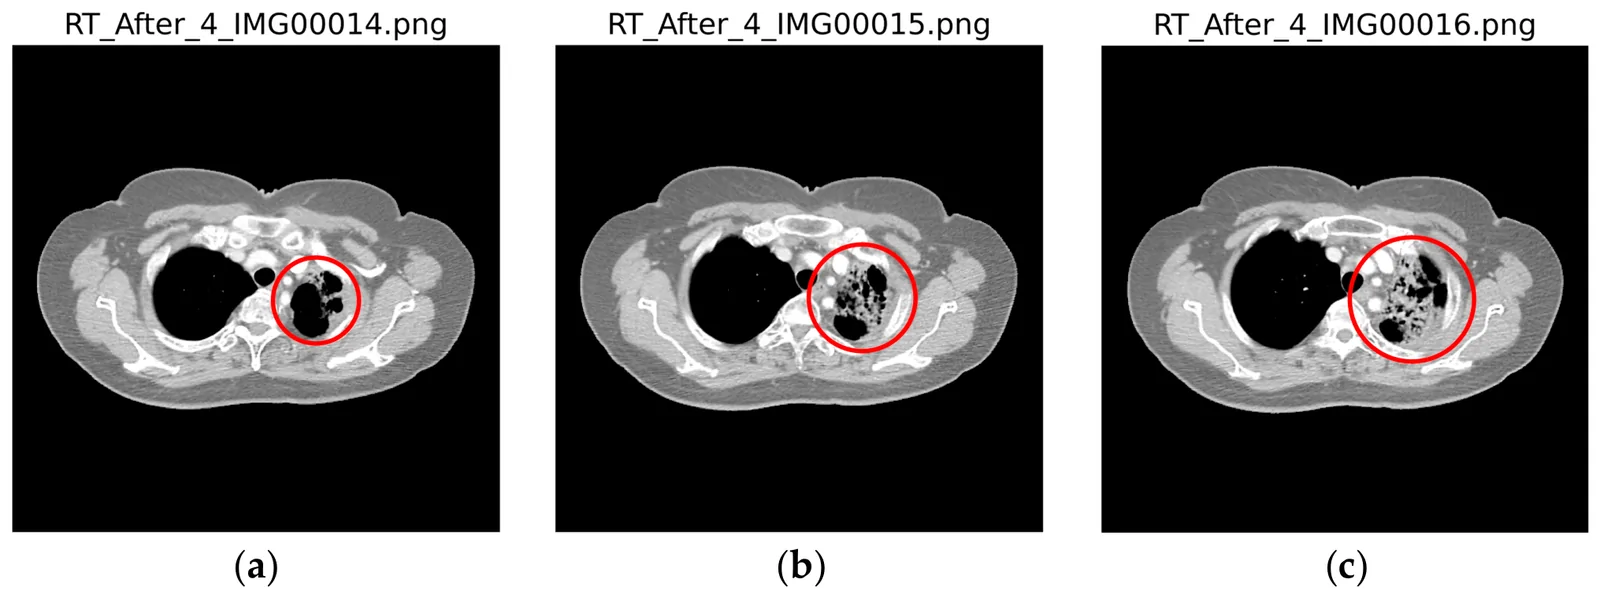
Representative RP-positive CT slices identified by the stacking classifier model. (a–c) are slices 14, 15, and 16, respectively, of patient No. 4. The red circles indicate suspected RP-related regions for qualitative visual reference. Abbreviation: CT: Computed Tomography.
